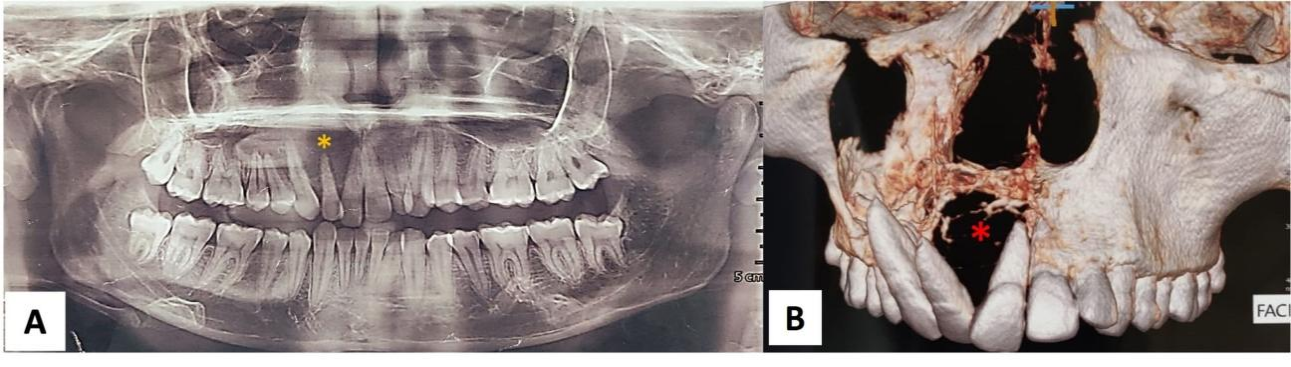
A: OPG reveals a radiolucent lesion (yellow asterisk) with displacement of the maxillary anterior teeth. B: CBCT reveals osteolytic lesion with displacement of the maxillary anterior teeth (red asterisk)
